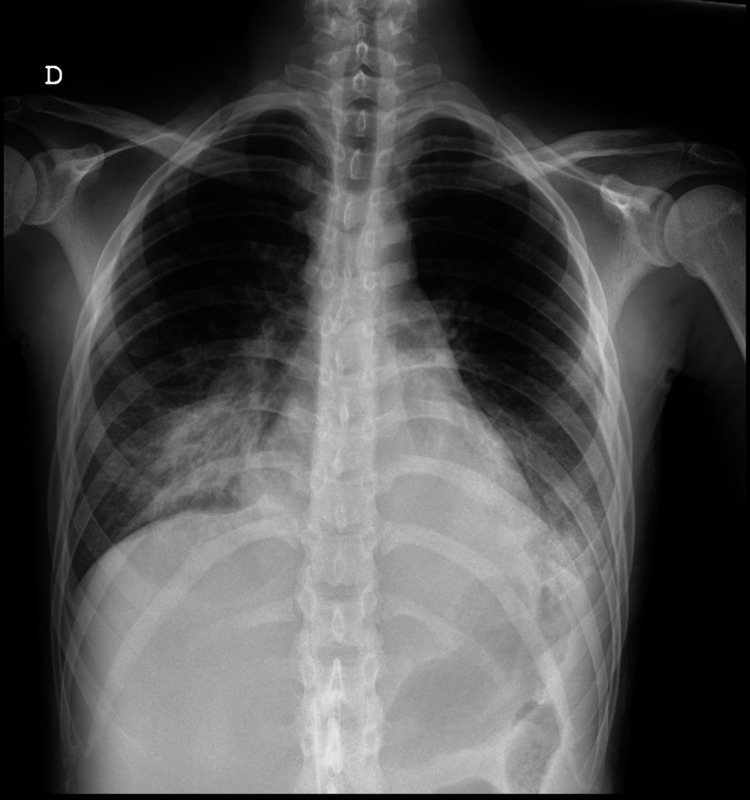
Chest x-ray with hypotransparency of the right lower lobe
